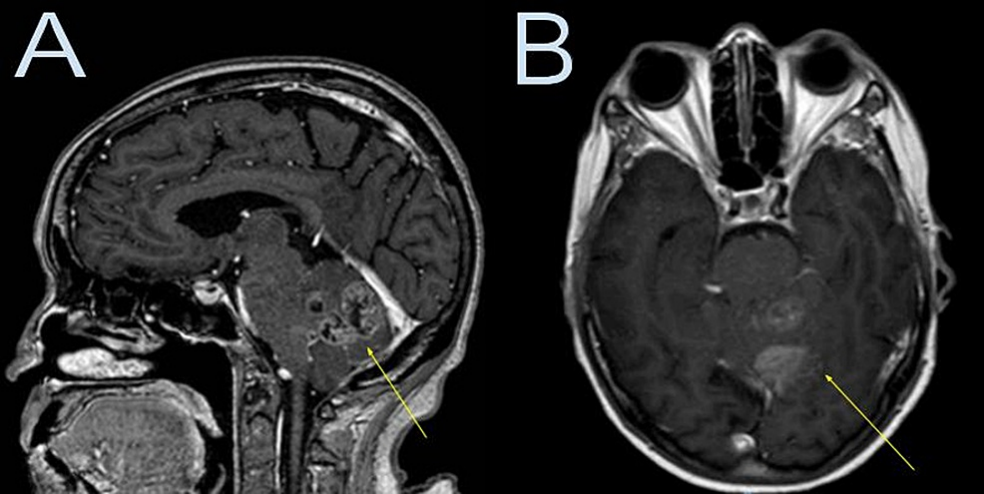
Brain MRI with contrast at the initial evaluation Brain MRI with contrast at the initial evaluation (A: sagittal, B: axial) shows a heterogeneously irregular peripheral rim enhancing solid and cystic, necrotic intra-axial infratentorial mass centered in the superomedial left cerebellar hemisphere (yellow arrow) with extension into the medial aspect of the right cerebellar hemisphere.
